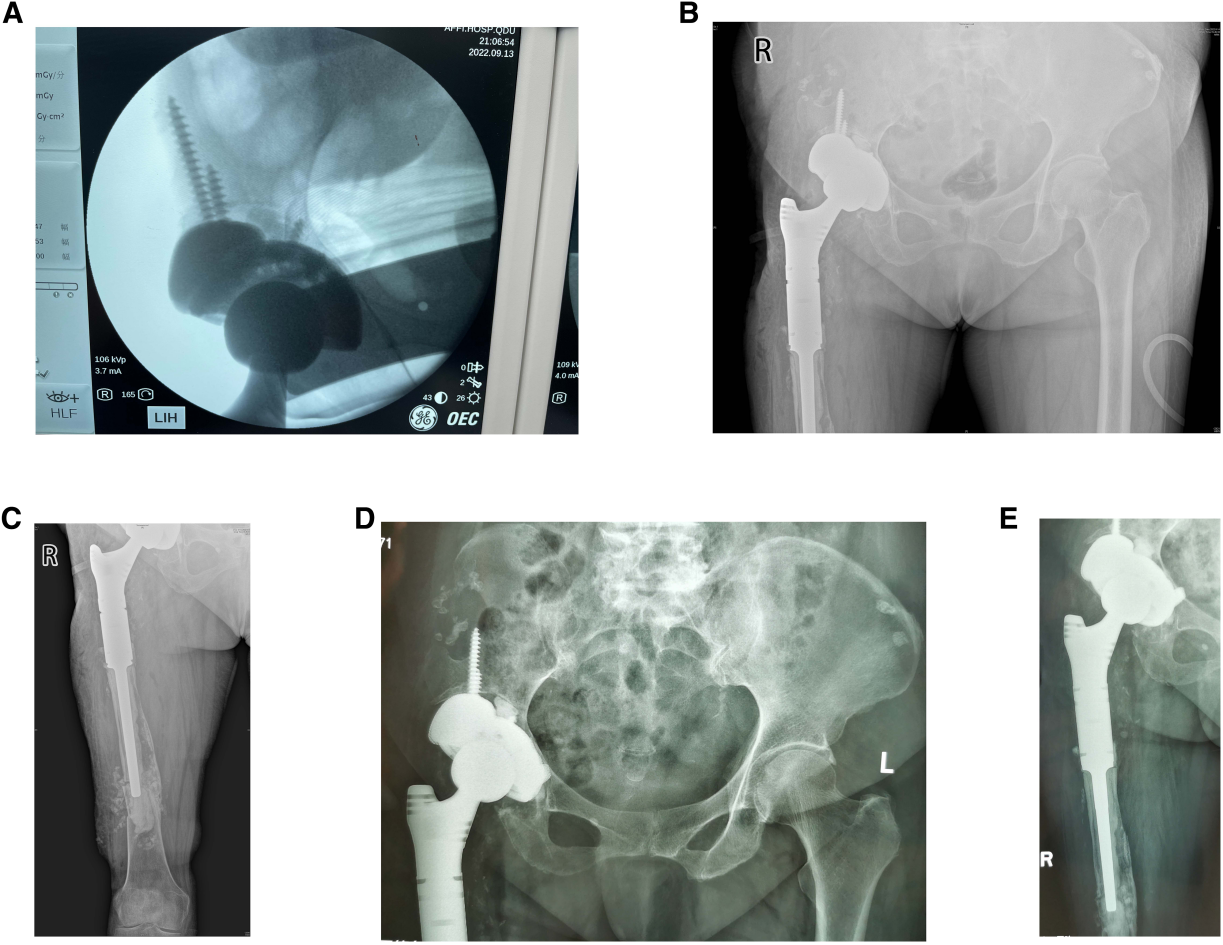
(A) Intraoperative C-arm radiograph showed a good position of augmented modules and prostheses. (B,C) Radiographs of the hip and femur were taken at the bedside 1 day postoperatively. (D,E) Radiographs of the hip and femur were taken at the outpatient clinic 5 weeks postoperatively.
